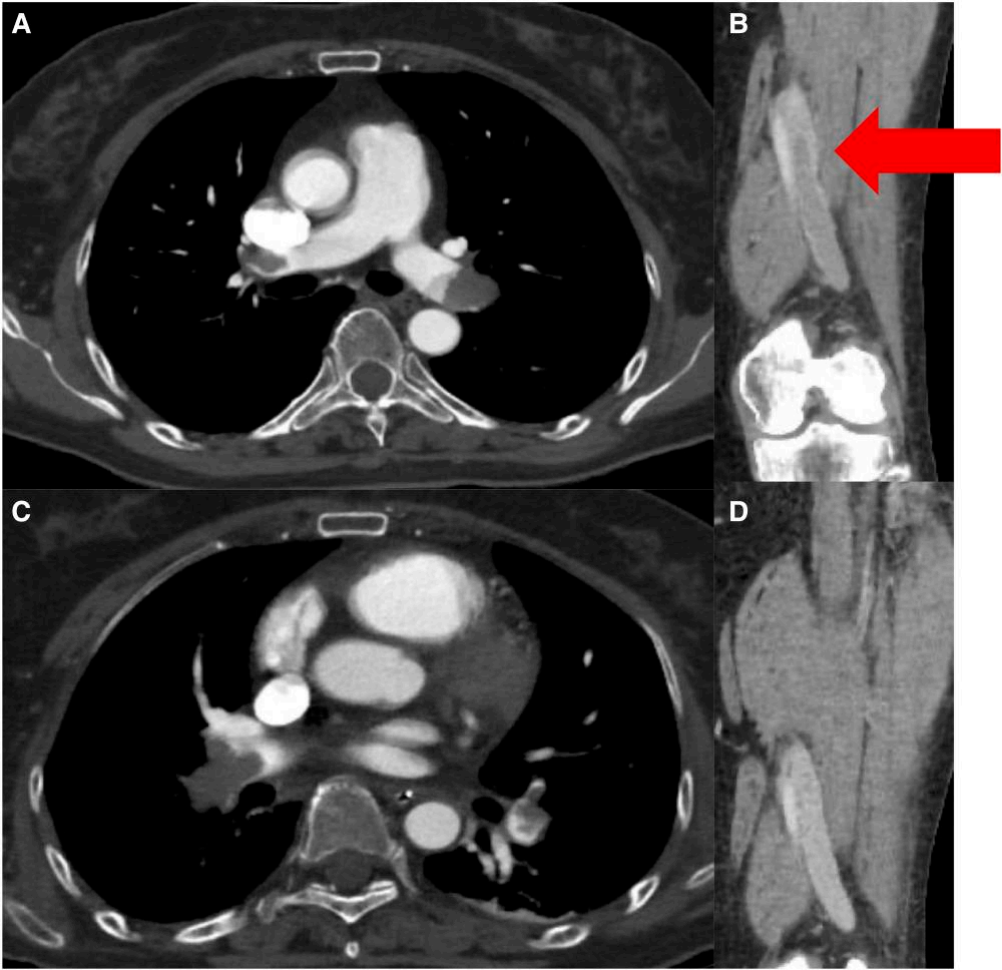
Time course of the pulmonary embolism and deep vein thrombosis (*A* and *B*) contrast-enhanced computed tomography reveals bilateral pulmonary embolism and deep vein thrombosis in the left superficial femoral vein (arrow). (*C* and *D*) Follow-up enhanced computed tomography demonstrates a large thrombus in the right pulmonary artery and resolution of the deep vein thrombosis in the left superficial femoral vein.
